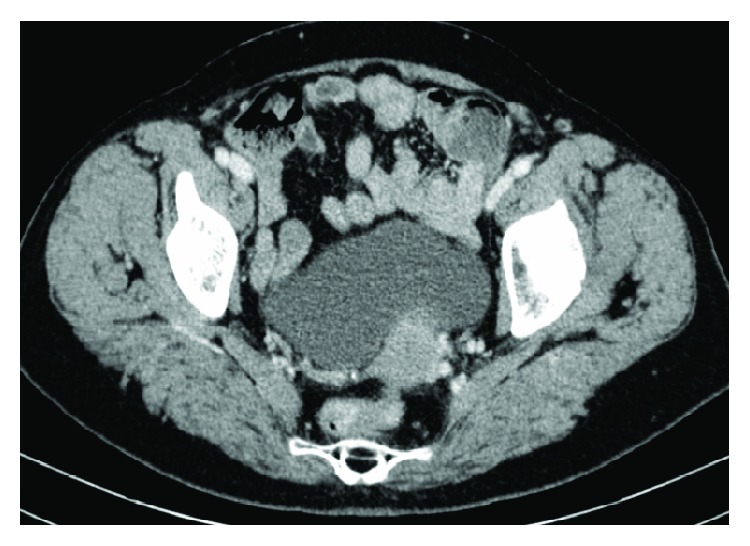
CT scan (no signs of lesions or intussusception).
